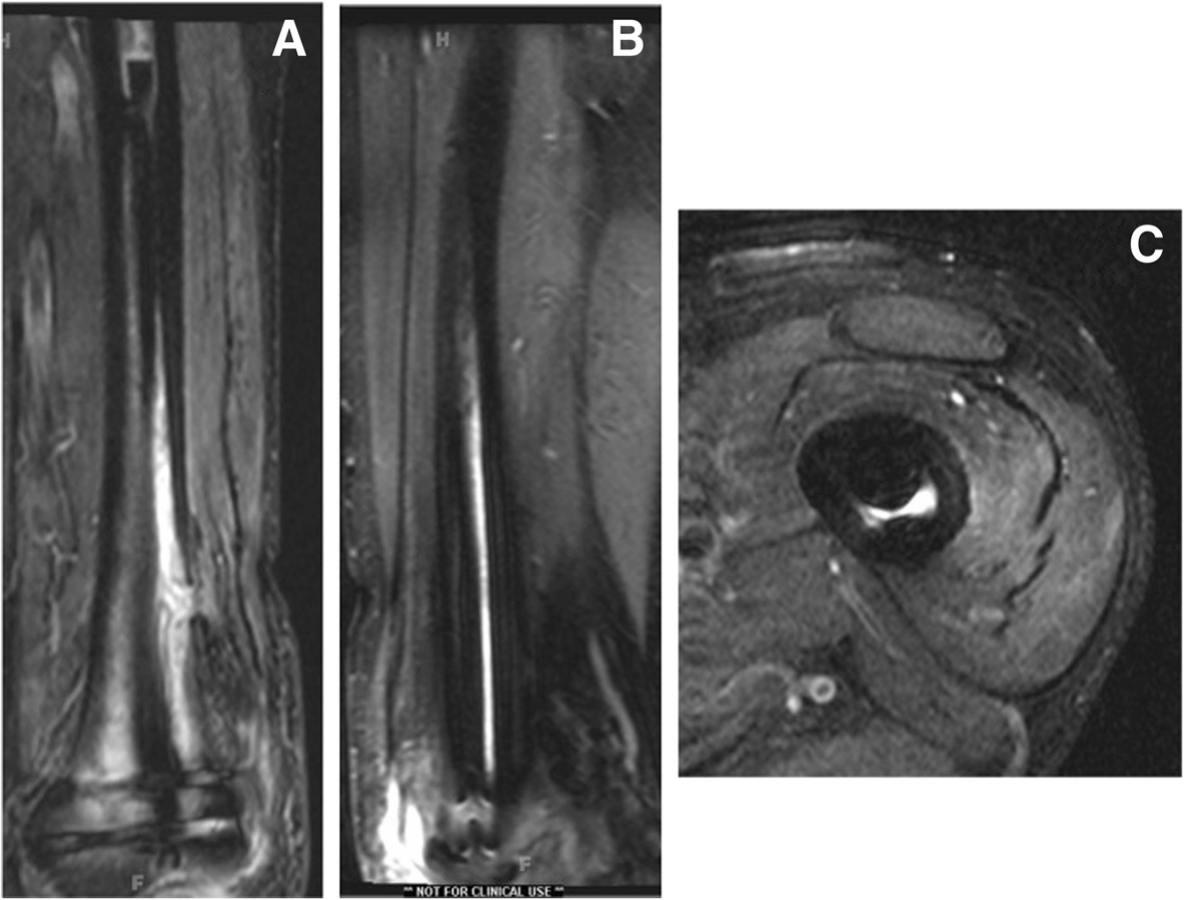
Follow up MRI scan with contrast at 8 weeks postoperative confirming overall reduction in size of osseous abscess and distal femoral enhancement on a coronal STIR (A), sagittal STIR (B) and axial STIR (C) of the distal femur.
